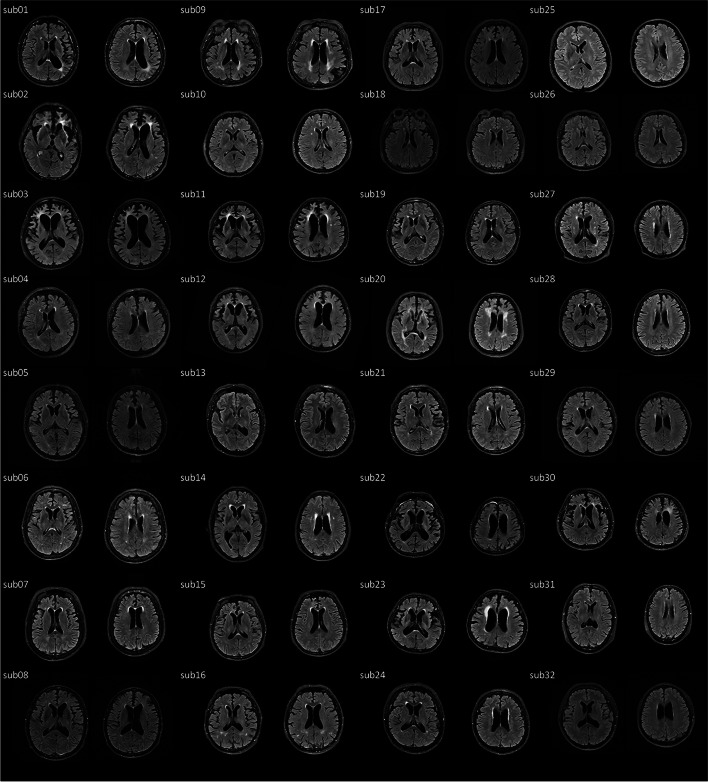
Raw images of white matter hyperintensity (WMH) in patients with FTD. In the raw images of 32 patients with FTD, the WMHs was observed in periventricular in T2 Fluid-Attenuated Inversion Recovery (FLAIR) images. It is more prominent in the anterior brain area, and some affect the posterior brain area
